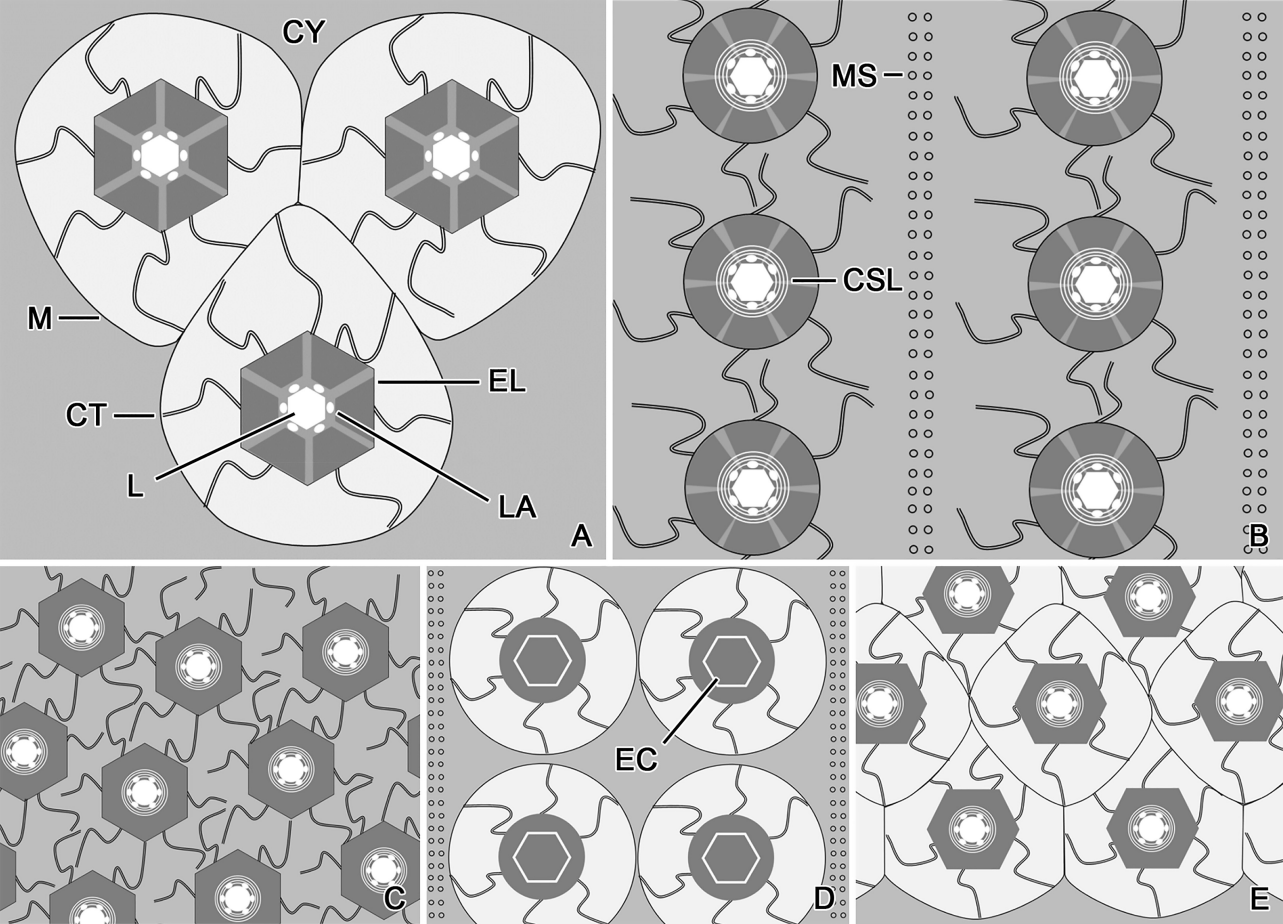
Schemes showing transverse sections of trichites and associated structures accompanying the girdle kinety in strombidiid (A–C), pelagostrombidiid (D), and tontoniid (E) oligotrichids as inferred from literature (B–E). Note that the trichites are not drawn to scale. (A) *Strombidium biarmatum* (this study). (B) *Strombidium inclinatum* and *Novistrombidium testaceum* (Modeo et al., 2001, 2003). The trichite membrane tightly surrounds the trichite proper, and the curved tubules extend in the cytoplasm. The trichite clusters are separated by microtubule sheets. (C) *Strombidium sulcatum* (Fauré‐Fremiet & Ganier, 1970). The trichite membrane tightly surrounds the trichite proper, and the curved tubules extend in the cytoplasm. (D) *Limnostrombidium viride* (Bardele et al., 2018). The curved tubules extend in the space between the trichite membrane and the trichite proper whose lumen is filled by an electron‐dense core. The trichite clusters are separated by microtubule sheets. (E) The secondarily tailless tontoniid *Laboea strobila* (Agatha et al., 2004; Jonsson, 1987). The curved tubules extend in the space between the trichite membrane and the trichite proper. CSL, concentric sheet layer; CT, curved tubules; CY, cytoplasm; EC, electron‐dense core; EL, electron‐dense layer; L, lumen; LA, lacunas; M, membrane; MS, microtubule sheets.
